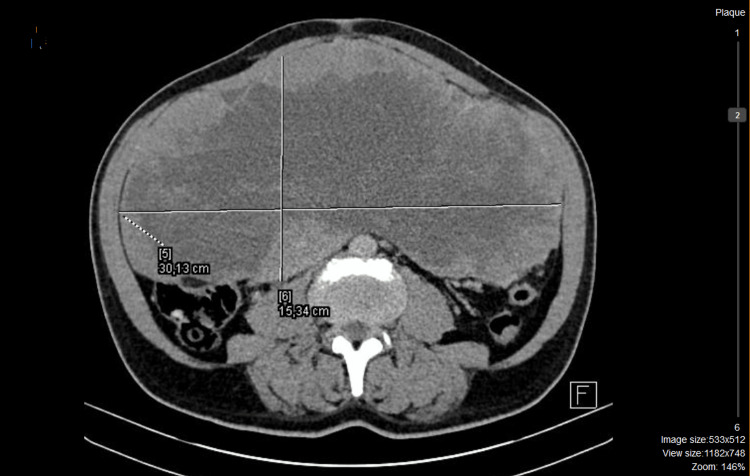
Abdominal computed tomography (CT) imaging revealing a 30x16x26 cm abdominal mass before treatment with imatinib (axial section).
